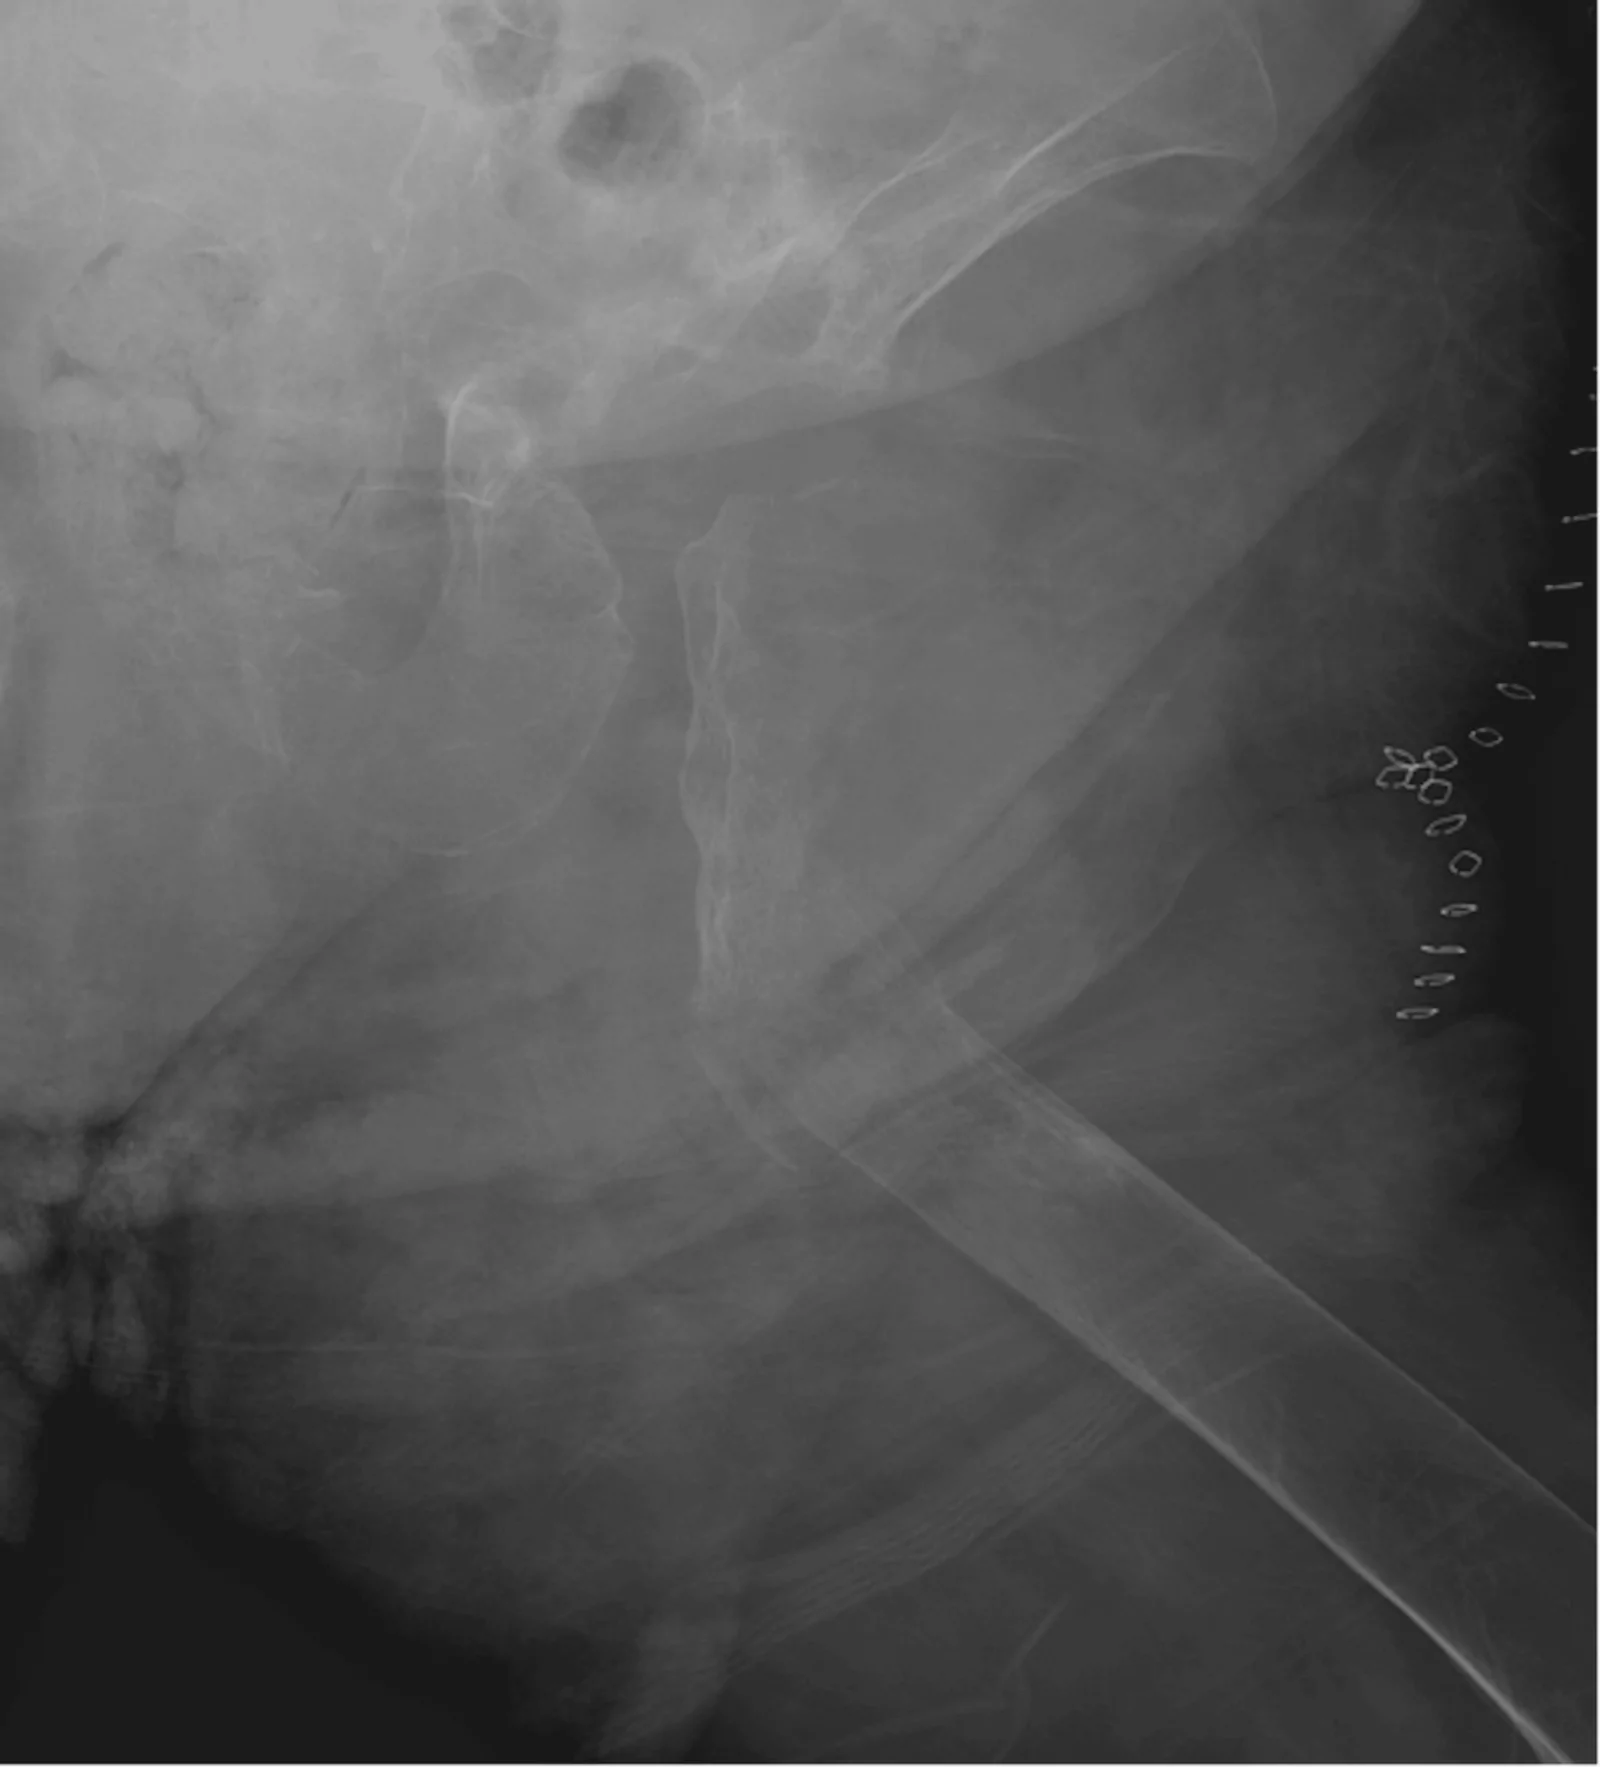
Anteroposterior (AP) radiograph of the left hip. The image reveals the removal of hip hemiarthroplasty hardware and osseous deformities of the left acetabulum and proximal femur.
